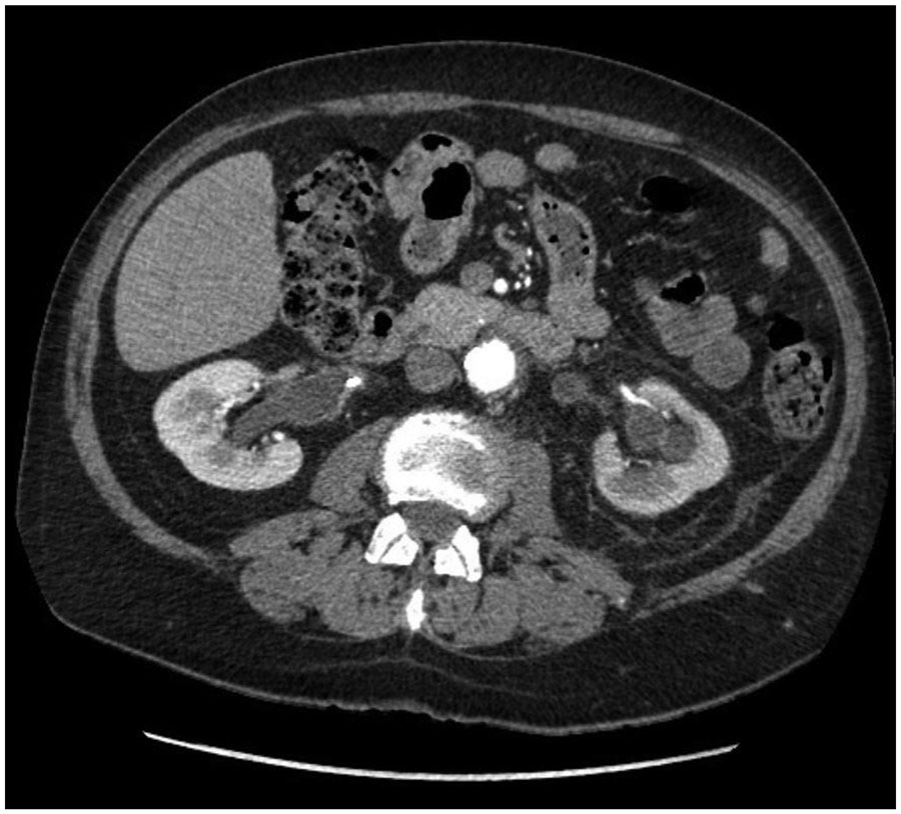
CT scan of subjects with urorenal subtype of retroperitoneal fibrosis. Bilateral hydronephrosis with inflammatory tissue involving ureter.
